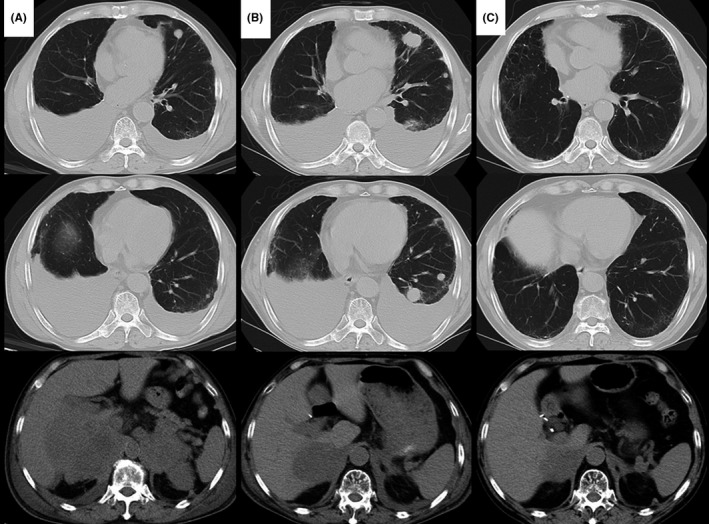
Computed tomography (CT) image during nivolumab therapy. A‐C, Before initiation, after 6 cycles, and after continuous administration of nivolumab therapy. CT showed that although the adrenal gland had shrunk without first increasing, the pleural effusion and pulmonary metastasis had increased once, and then shrunk
